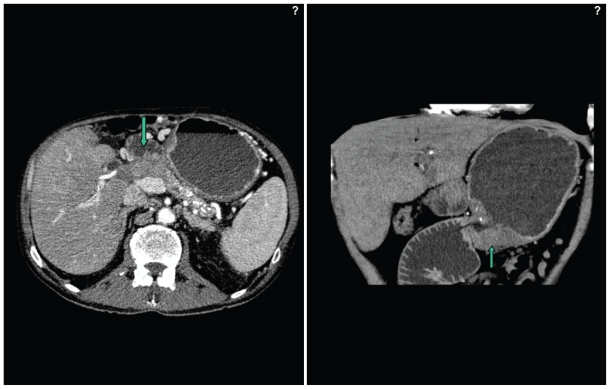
Abdominal CT demonstrating the large, distended fluid-filled gastric remnant, obstructed by a mass at the anastomotic site (arrow) consistent with malignant neoplasm. Disease recurrence of ductal adenocarcinoma of the pancreas was suspected.
